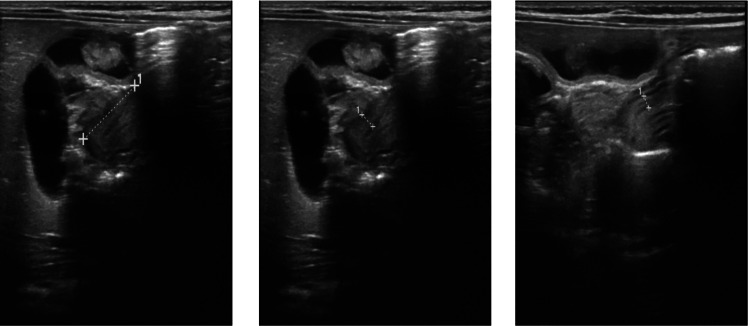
Ultrasound pylorus with a thickness of 3 mm, length of 13 mm, and diameter of 15 mm. Gastric emptying was noted with feeding.
